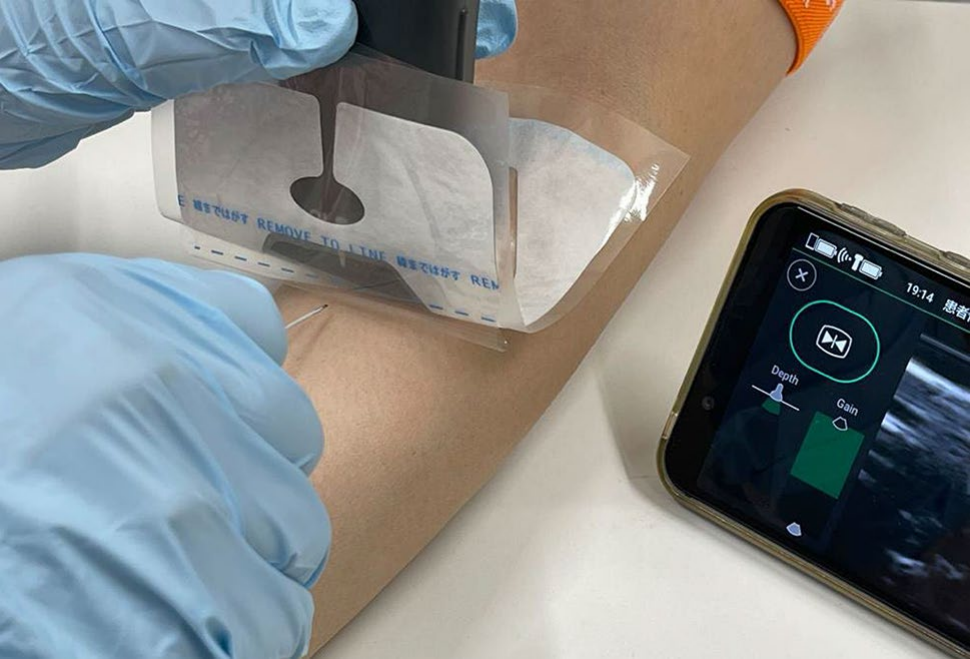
The scene of performing a puncture using film for ultrasound-guided puncture. A sterile film is placed between the probe and the needle, preventing direct contact between them. Since the film is sterile, even if the needle accidentally touches the film, it will not compromise sterility
